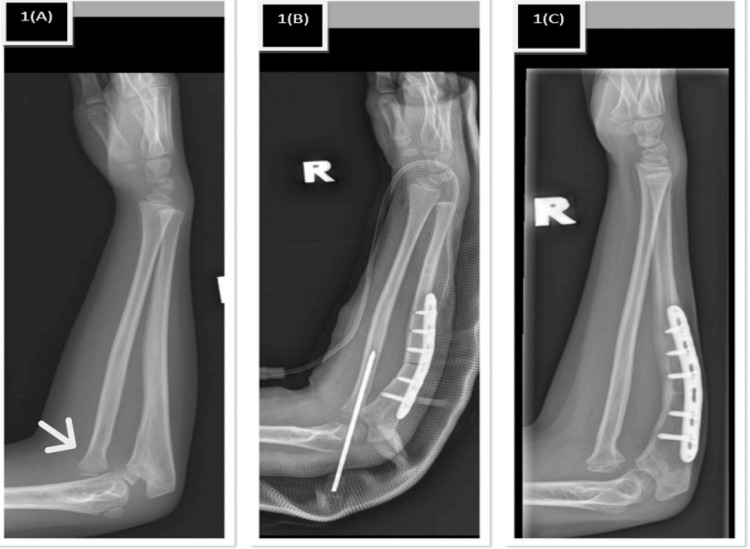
Plain radiograph of patient 1. (1A) Bado type 1 with plastic deformation of the ulna. (1B) Post-ulna transverse osteotomy with transcapitellar K-wire fixation of the radial head. (1C) Anterior radial head redislocation after K-wire removal with united ulnar osteotomy.
